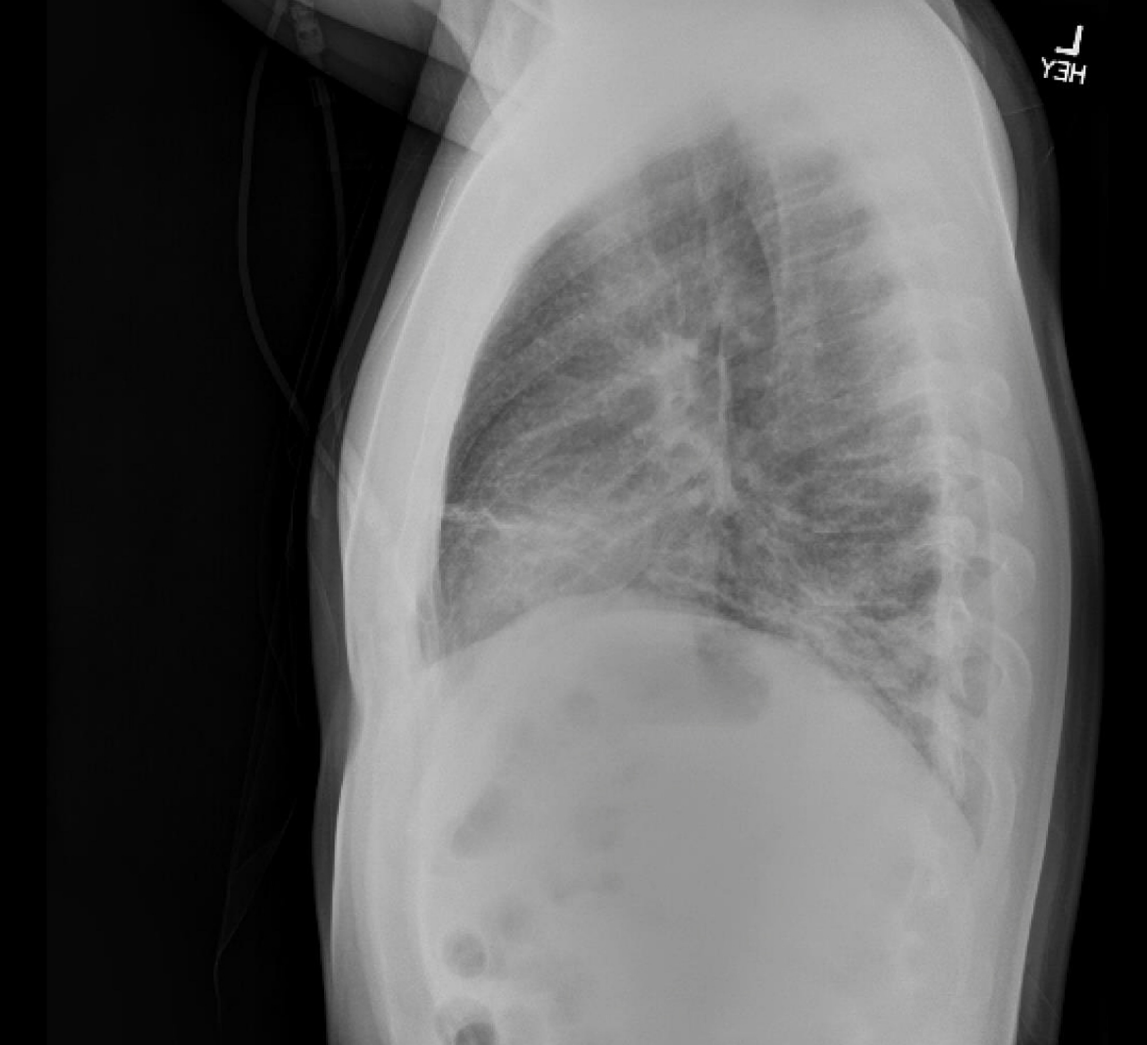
Chest X-ray in the emergency department (lateral view). Image shows increased interstitial markings with suggestion of a right lower lobe more focal consolidation.
